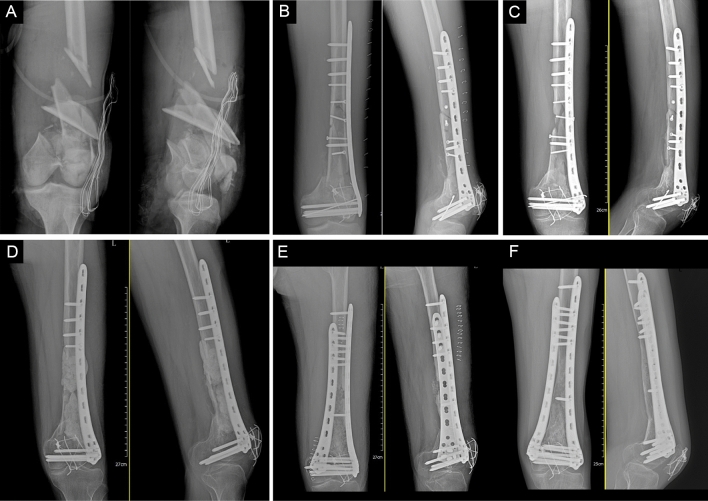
a Anteroposterior and lateral radiographs of the left femoral of a 20-year-old male injured in a car accident. The patient experienced an open comminuted fracture of the distal femur. b Anteroposterior and lateral radiographs of the left femur after lateral plate. c Anteroposterior and lateral radiographs of the left femur after half a year. Unfortunately, a localized infection developed and formed a sinus tract, and the fracture did not heal. d Anteroposterior and lateral radiographs of the left femur after bone debridement and placement of a polymethylmethacrylate spacer in the femoral bone defect. e Anteroposterior and lateral radiographs of the left femur after bone grafting. f Anteroposterior and lateral radiographs of the left femur taken 17 months postoperatively show a mature bone callus
